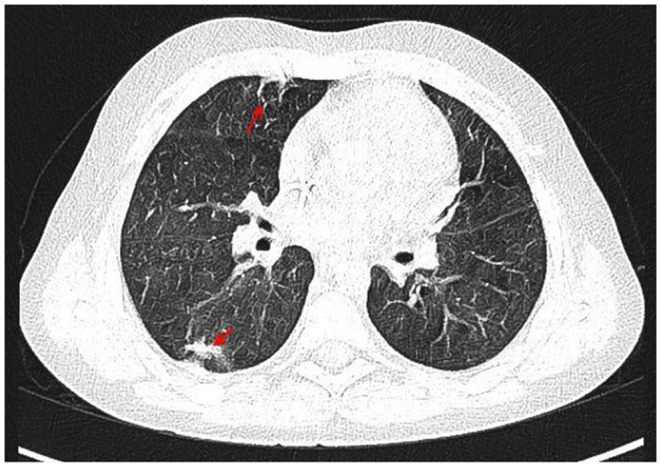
CT of the chest with contrast medium, arterial phase, coronal view. Irregular, thickened peribronchium with narrowing of the lumen of segmental and subsegmental bronchi, fibrosis (marked with arrows) with compensatory dilation of the bronchial lumen (bronchiectasis), signs of bronchiolitis in PS10 and LS 10, irregular nodules in LS9 and LS10, a trace of fluid in the pericardium, enlarged right upper paratracheal, below carina, paraaortic, perivascular lymph nodes.
